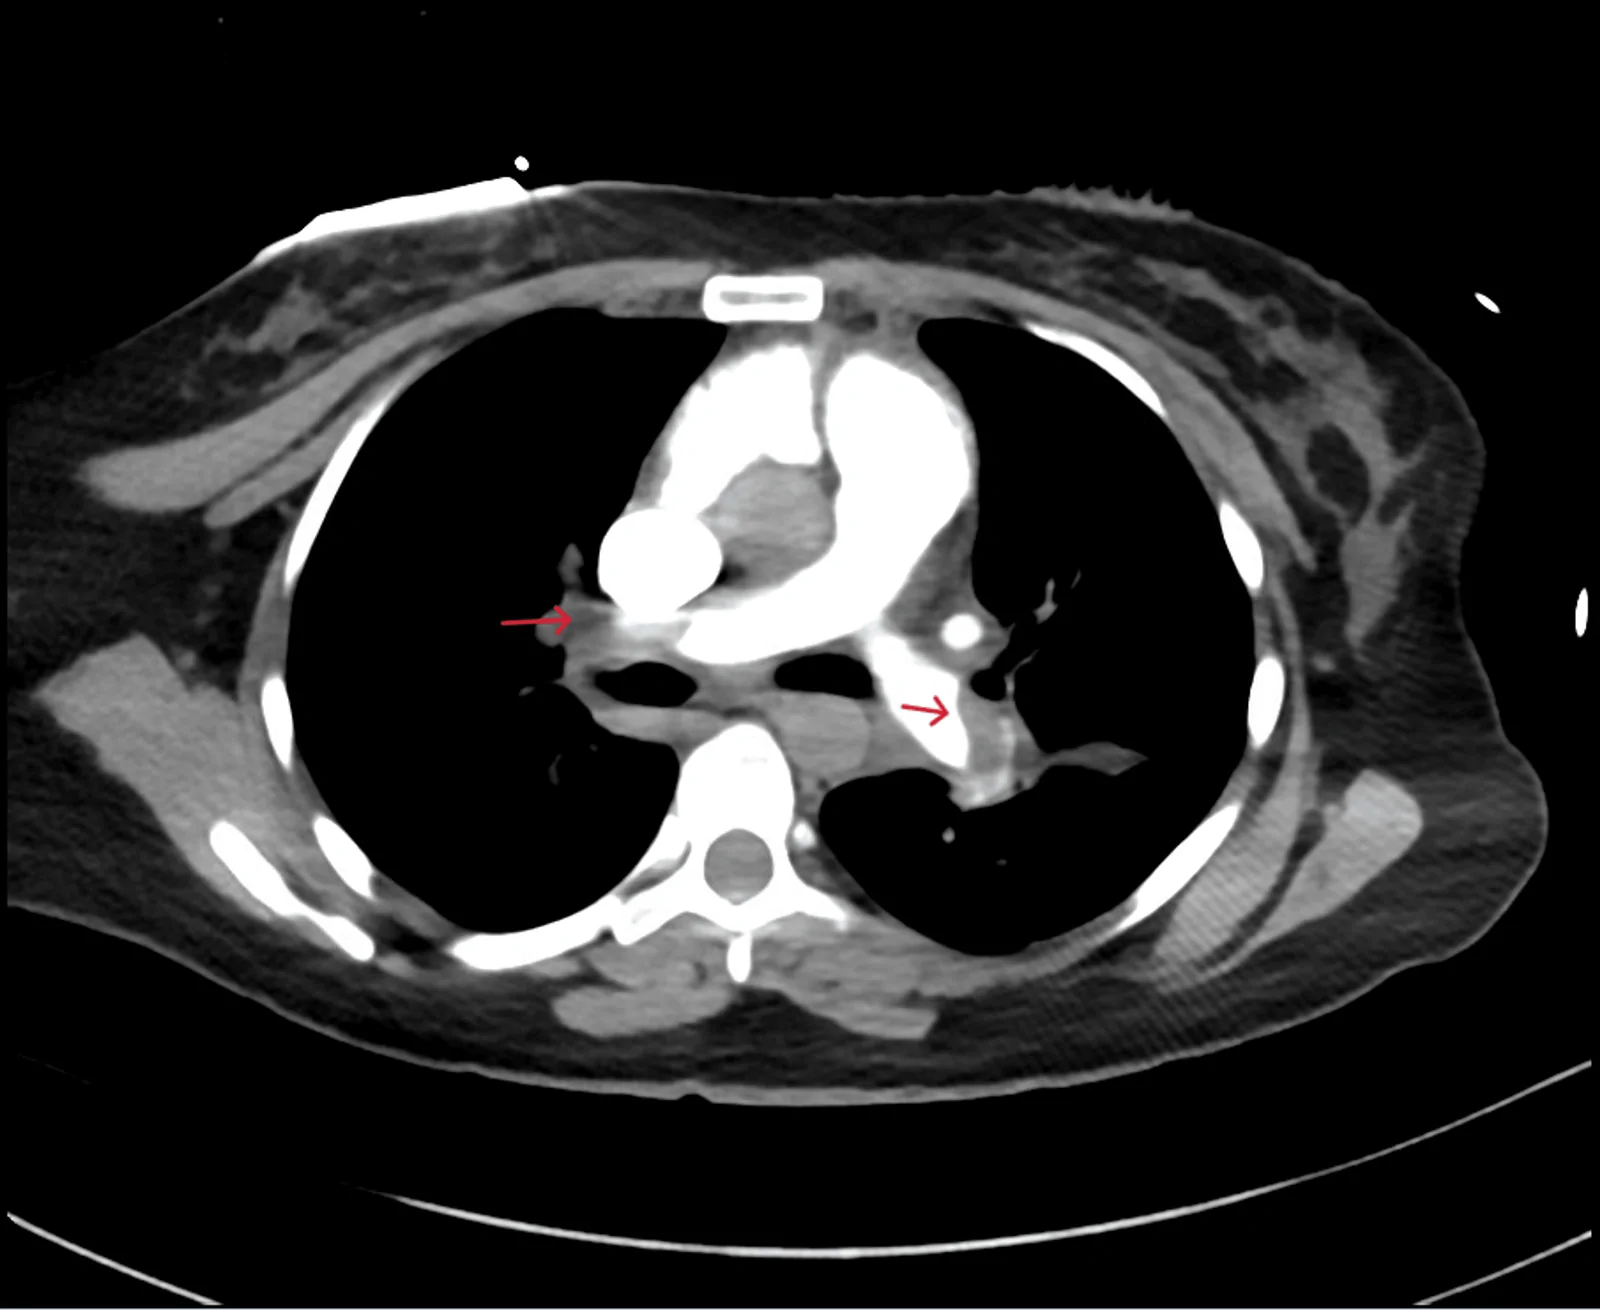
Computed tomography pulmonary angiography (CTPA) demonstrating bilateral filling defects within the right and left main pulmonary arteries (red arrows)
